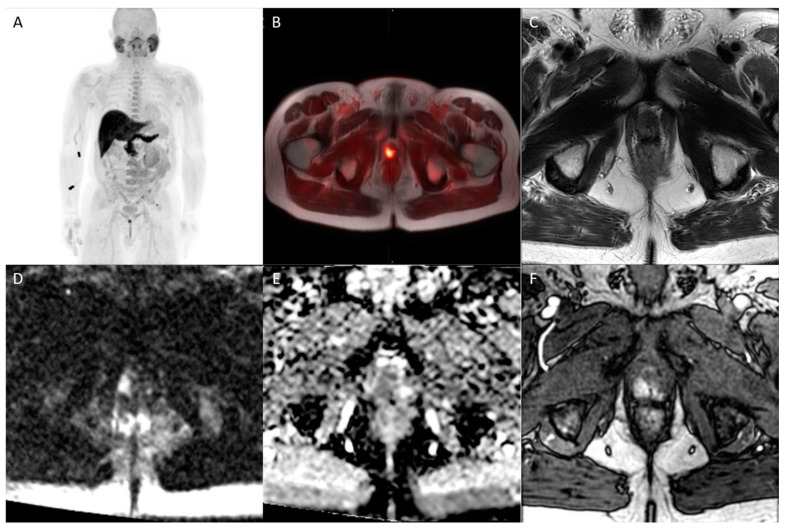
Whole-body maximum intensity projection (A), axial fused fluiclovine-PET/MRI (B), axial T2 (C), diffusion-weighted b2000 (D), apparent diffusion coefficient (E), and dynamic contrast-enhanced (F) images demonstrating focal uptake of radiotracer in the right prostatic apex peripheral zone with corresponding MR defined T2 hypointense lesion with associated restricted diffusion and focal asymmetric enhancement (PI-RADS 5), consistent with prostate cancer.
